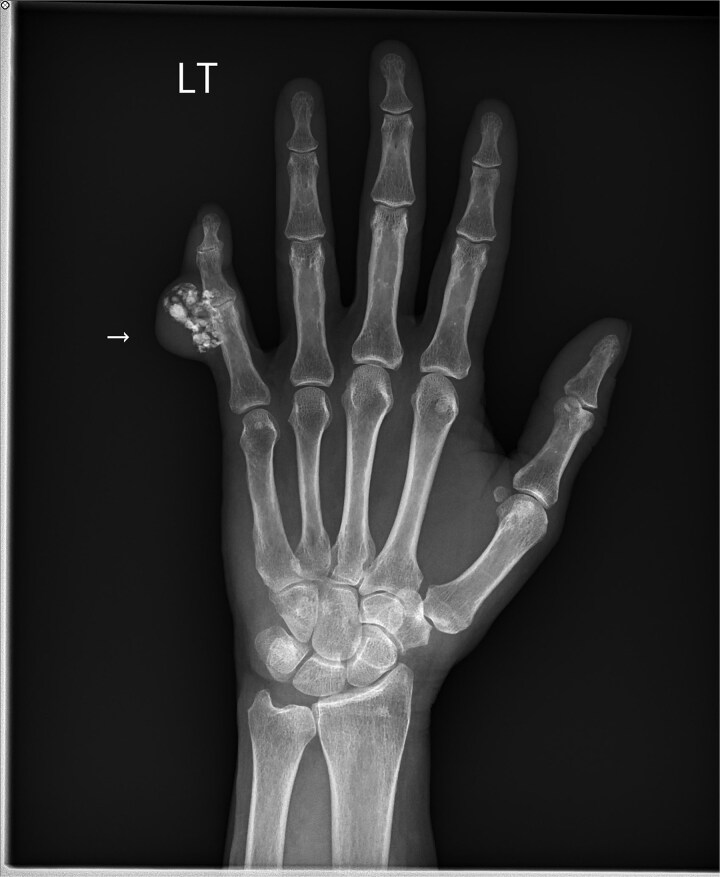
Plain radiographs demonstrated a 2 × 2 cm ovoid, partially calcified soft-tissue lesion.
